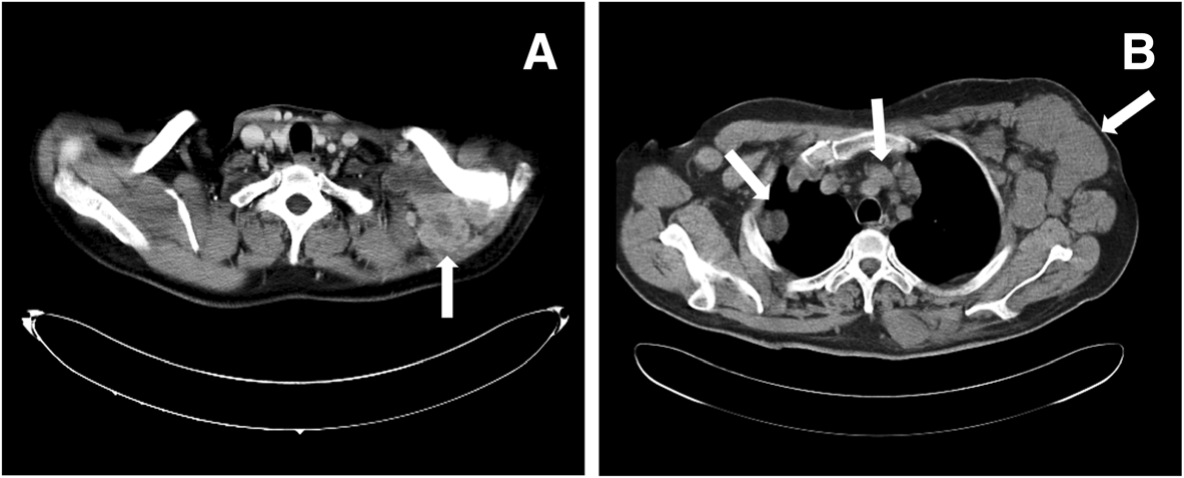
CT shows multiple fused nodular masses in the left supraclavicular fossa with necrosis 17 months after surgery (a, arrow). Subsequently, multiple nodules in the bilateral axilla, lungs and mediastinum are found 27 months after operation (b, arrows)
